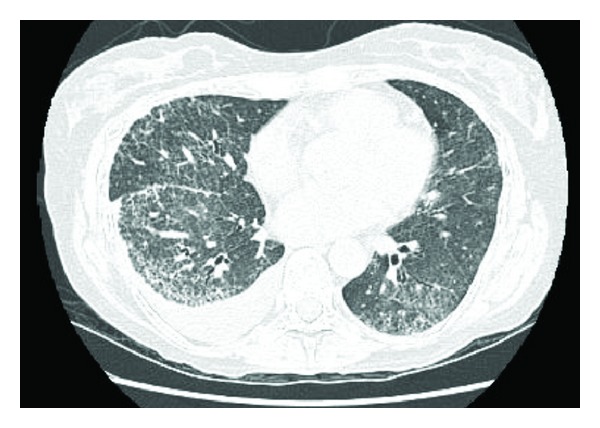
HRCT showing UIP (followup scan, May 2008).
